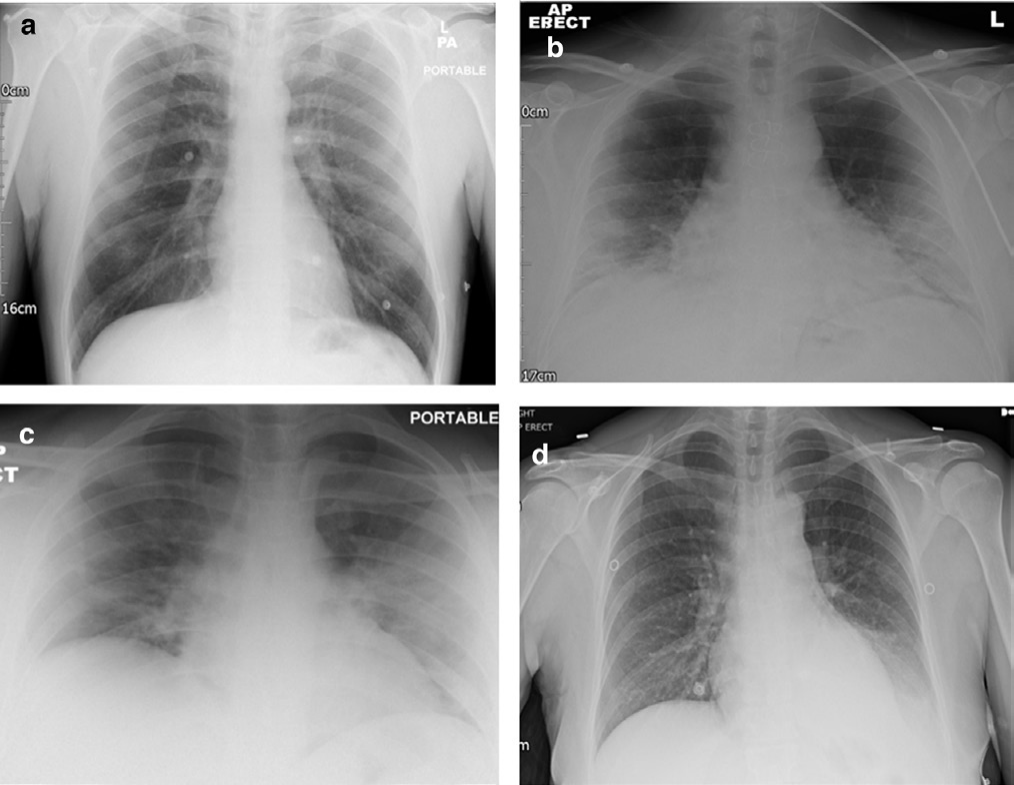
Example chest radiographs for the report categories. *A* = normal, *B* = indeterminate, *C* = classic/probable COVID-19, *D* = non-COVID-19
